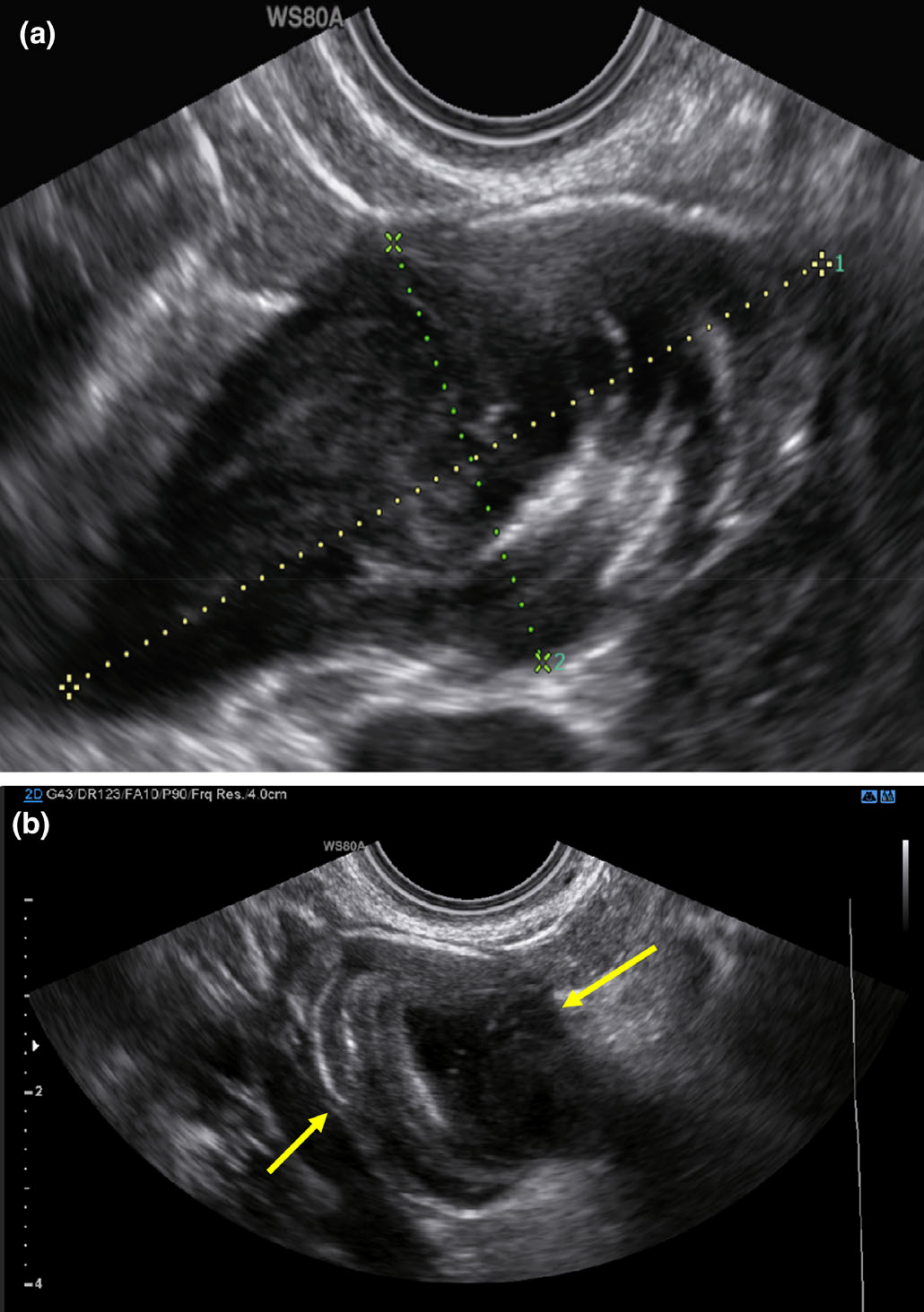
Transvaginal ultrasound scan showing hyperechoic concentric lines representing mucin deposition of the low grade appendiceal mucinous neoplasm (LAMN) at 6 weeks of pregnancy. The mass measured 60 millimetres (a) by 30 millimetres (b).
